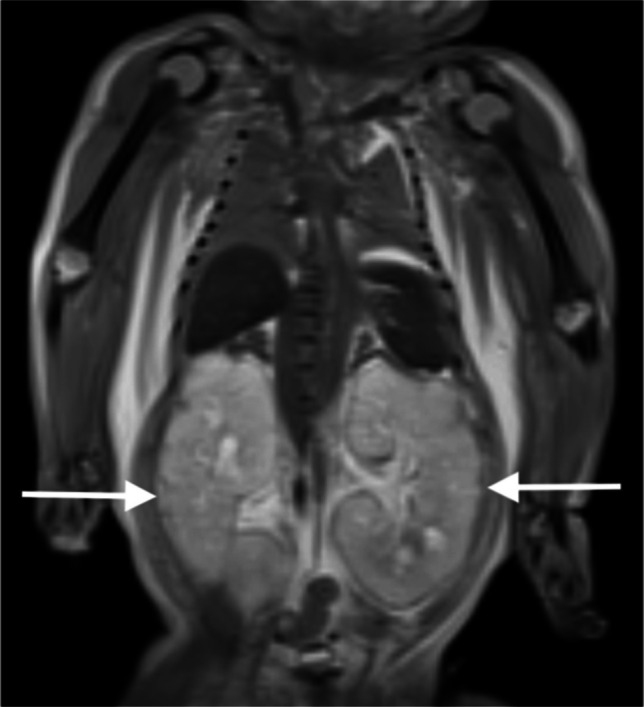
Coronal T2-weighted postmortem magnetic resonance image in a 33-week-old male fetus showing symmetrically enlarged kidneys (*arrows*) in the setting of autosomal recessive polycystic kidney disease. Adapted with permission [33]
